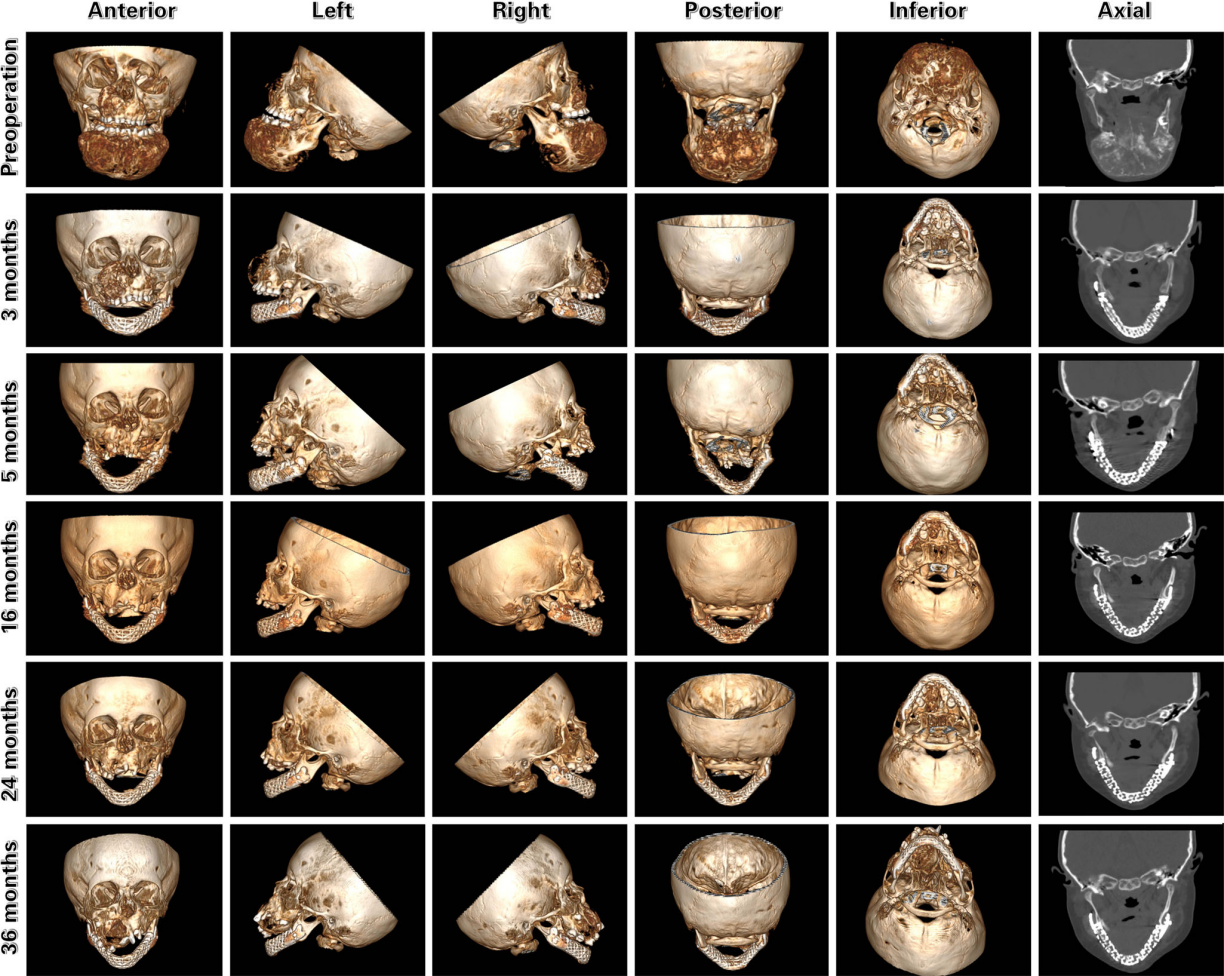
Spiral CT scanning following up on case 1. The radiological images indicated that no displacement, loosen or fracture of scaffold occurred, and mineralization of tissues was detected in the scaffold concentrated at the chin and the distal area of the scaffold.
